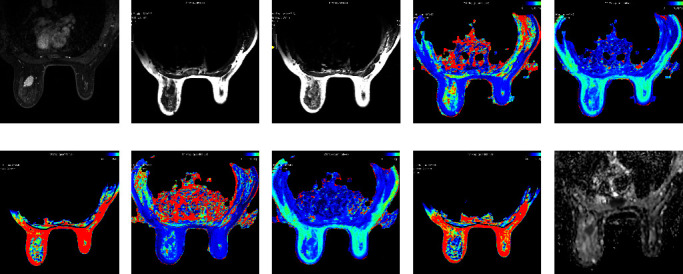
47-year-old female with invasive ductal carcinoma (IDC) of the left breast. Ki-67 expression was 20%. Axial contrast enhanced T1-weighted image (T1WI) showed an irregular homogeneous enhancing mass with circumscribed margin (a) The quantitative parameters were measured on SyMRI-T1WI before ((b) pre-T1 = 1495 ms, pre-T2 = 77 ms, and pre-PD = 80.8 pu) and after ((c) post-T1 = 683 ms, post-T2 = 69 ms, and post-PD = 67.4 pu) administration of the contrast medium. Similar images are shown for the mass on precontrast ((d) T1 mapping, (e) T2 mapping, and (f) PD mapping) and postcontrast ((g) T1 mapping, (h) T2 mapping, and (i) PD mapping) enhanced mapping images. The apparent diffusion coefficient (ADC) value was 0.936 × 10^−3^ mm^2^/s on the ADC map image (j).
